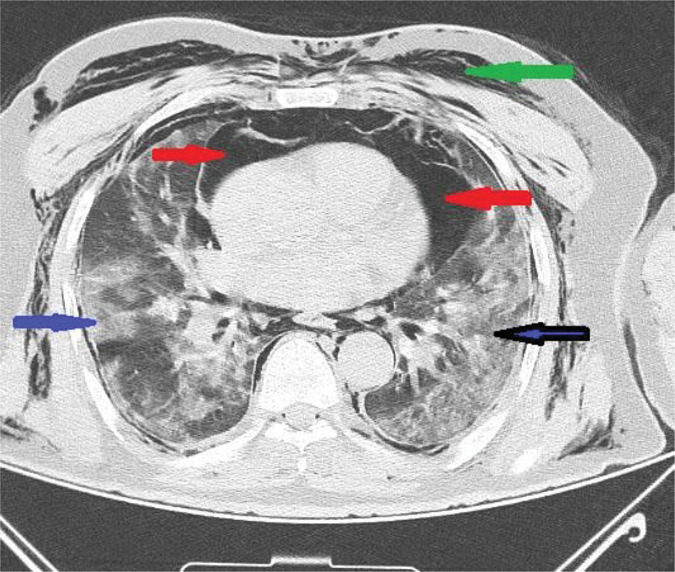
Thorax computed tomography shows diffuse air density around the heart (red arrow), ground glass appearance in bilateral parenchyma (blue arrow) and diffuse air entrapment under the skin (green arrow).
